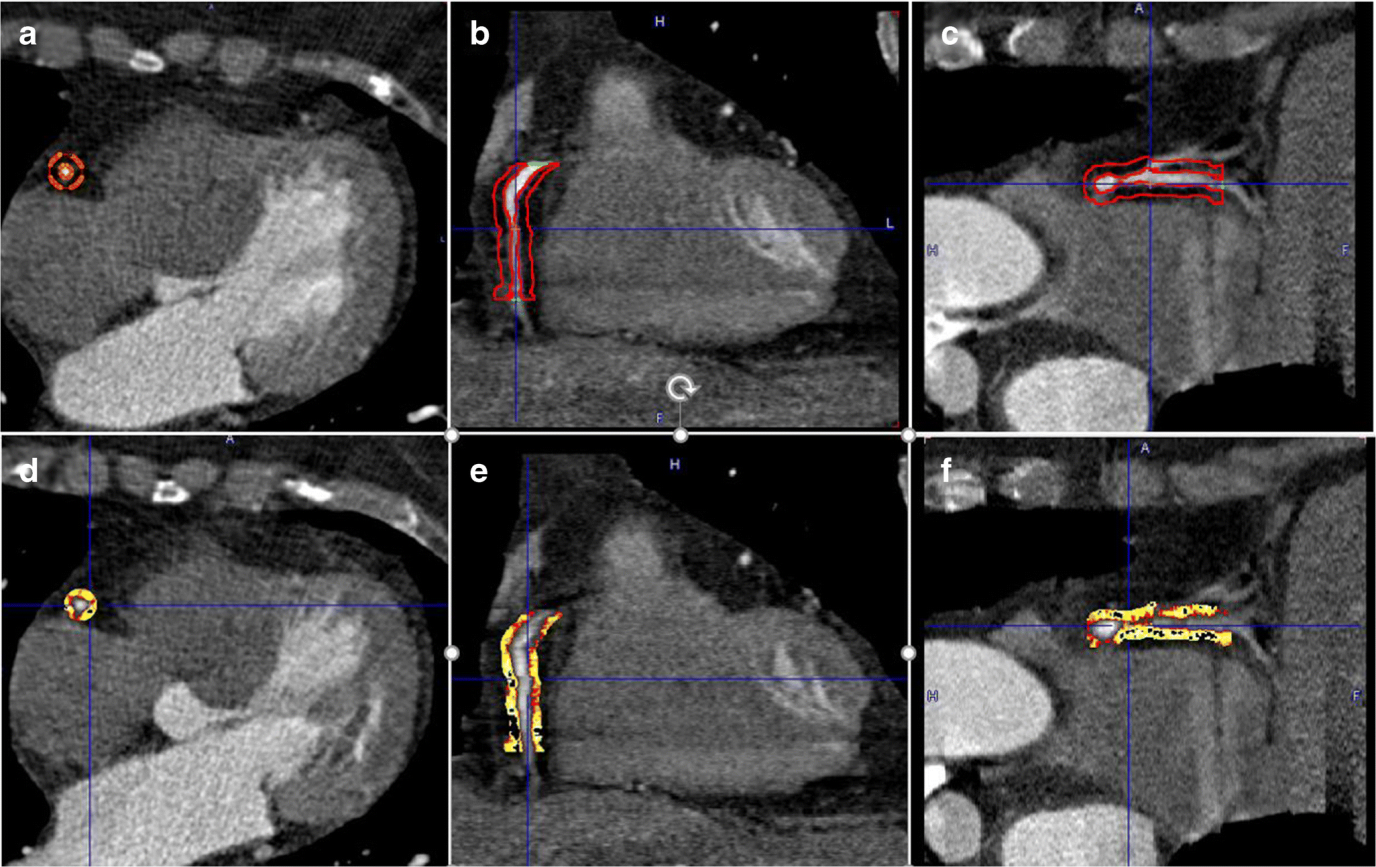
Perivascular fat attenuation index (FAI) phenotyping from coronary computed tomography angiography (CCTA) in the proximal 10–50 mm of the right coronary artery (RCA). a–c Volume of interest (VOI) in transaxial, coronal, and sagittal views. d–f FAI phenotyping in transaxial, coronal, and sagittal views
